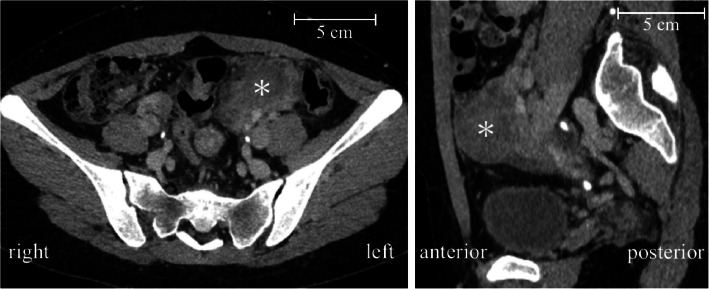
Preoperative abdominal computed tomography images. Left paraovarian cyst torsion (*) is visible in the coronal pelvic image (left). The sagittal abdominal image (right) shows the cyst (diameter 34 × 43 mm)
